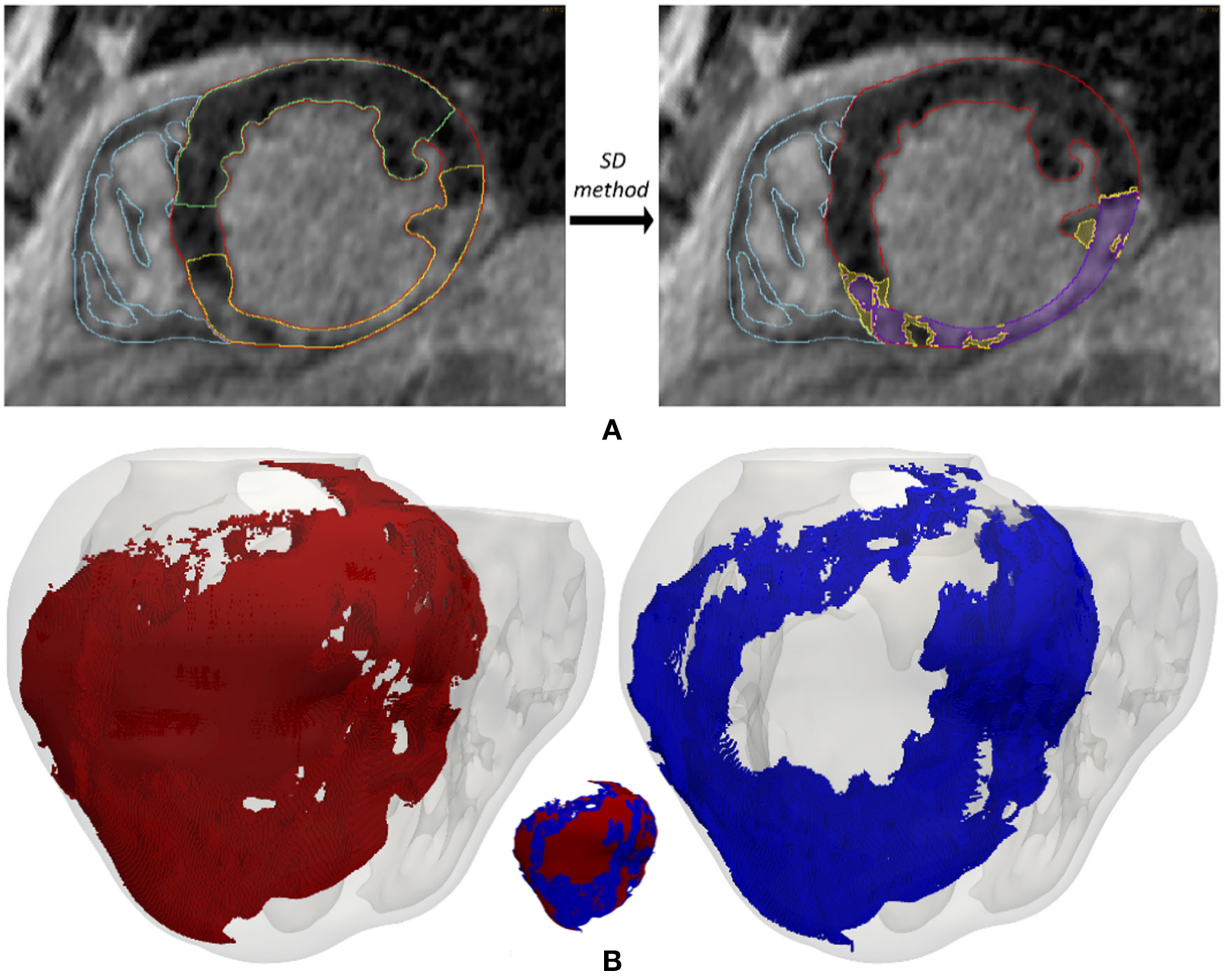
(A) Segmentation process from short-axis DE-MRI slices of the heterogeneous remodeling caused by MI, including infarct scar and BZ. Left panel shows the first step that involves the manual delineation of remote ROI (region-of-interest) (*green*) and infarcted ROI (*yellow*) within the LV myocardium ROI (*red*), also displaying the RV myocardium ROI (*cyan*). Right panel shows the segmentation for infarct scar (*purple*) and BZ (*yellow*) resulting from the application within the infarcted ROI of thresholds determined by SD method from the remote ROI of each slice. (B) 3D representation of the MI reconstructed from DE-MRI images, showing posterior views of the 3D surface model of ventricles (rendered with transparency) along with those hexahedral elements of volume mesh labeled as infarct scar (*red*) and BZ (*blue*).
